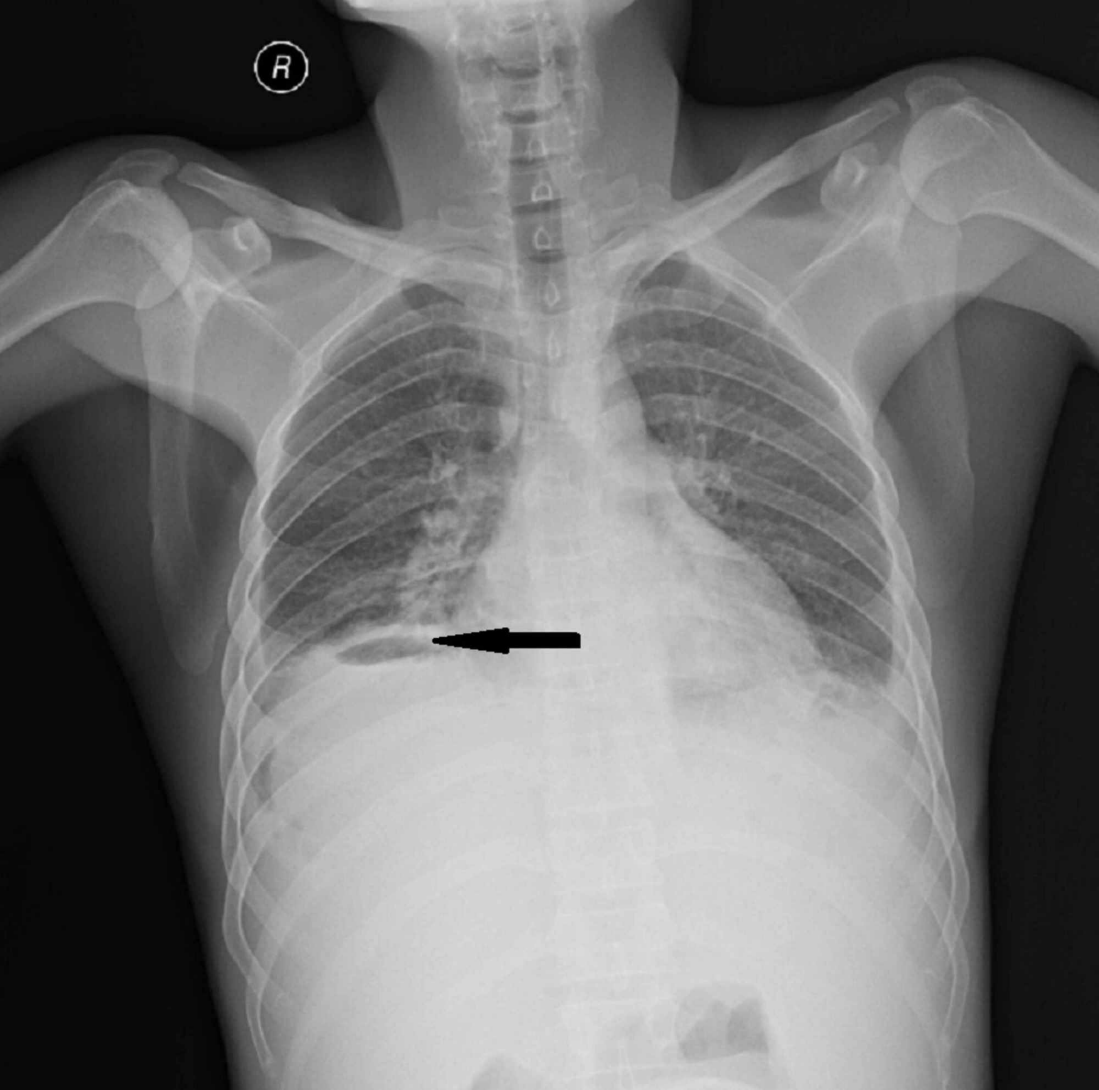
Erect chest X-ray showing free air under the diaphragm (arrow), a feature of bowel perforation.
